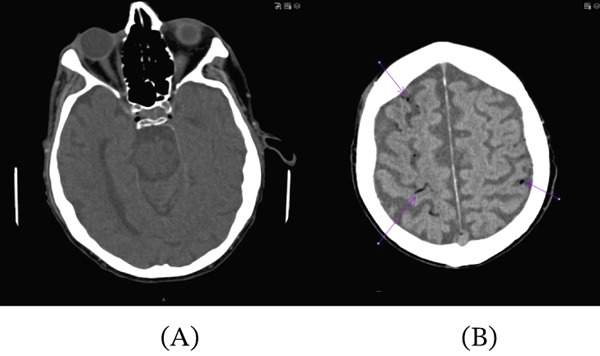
Computed tomography (CT) of the brain, adjusted towards lung window (A and B). Reveals air bobbles on both sides of the cavernous sinus (A) and shows the small air emboli (purple arrows in B) involving the leptomeningeal venules and arterioles along the subarachnoid spaces on both cerebral hemispheres.
